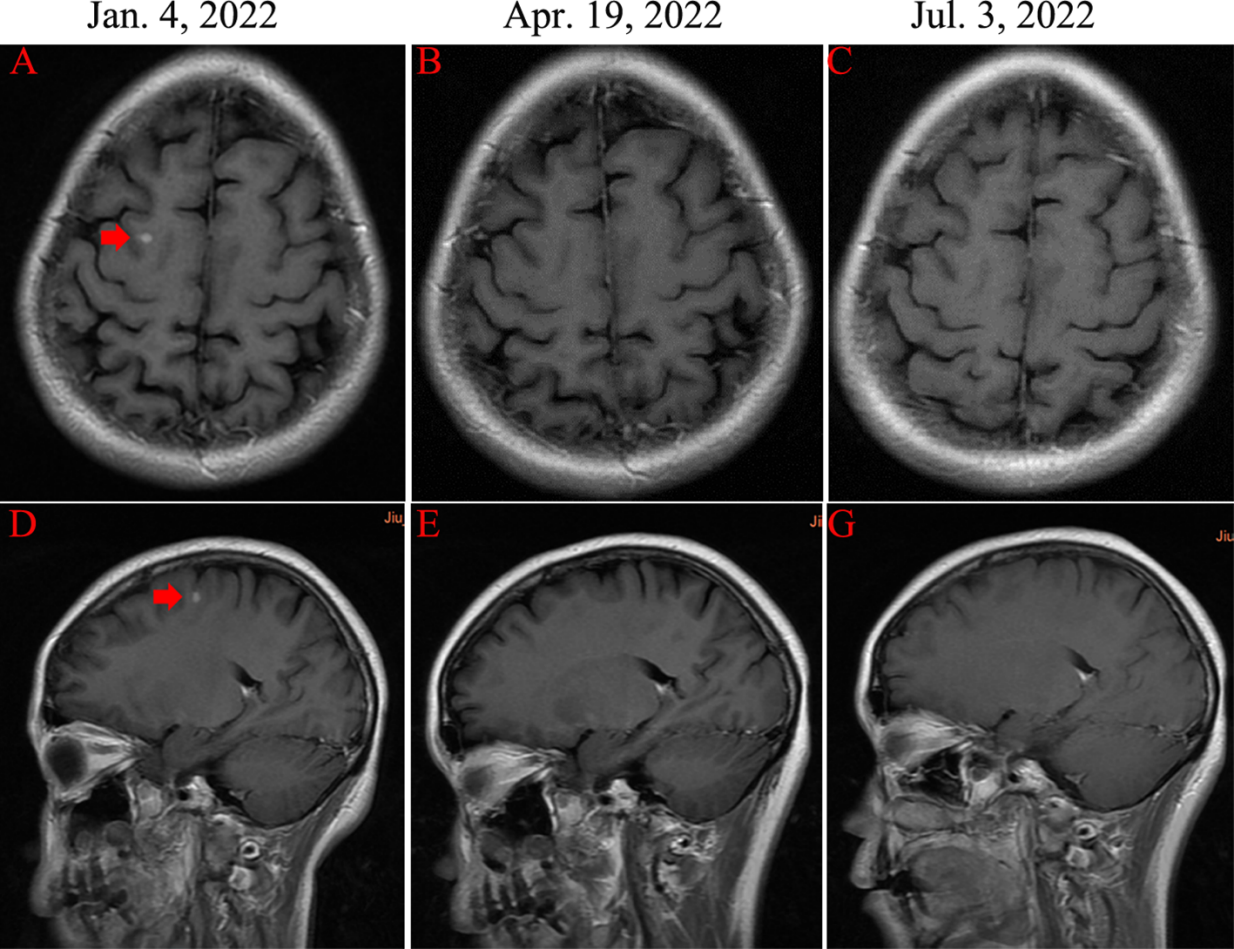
Magnetic resonance imaging (MRI) examinations of right frontal lobe metastasis (red arrow). (A, D) The presence of solid brain metastasis (diameter of 1 cm). (B, E) and (C, G) The disappearance of brain metastasis.
